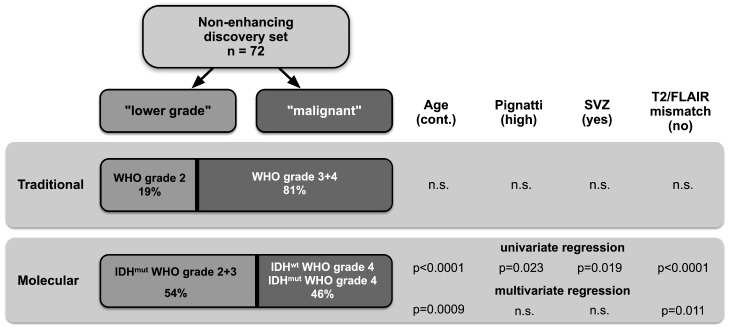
Malignancy was stratified by two different grading systems: (1) traditional grading (WHO grade 2 vs. WHO grade 3 + 4) and (2) molecular grading (IDH^mut^ WHO grade 2–3 vs. IDH^wt^ GBM WHO grade 4 + IDH^mut^ astrocytoma WHO grade 4). Molecular diagnostics allowed clinical and radiographic features to predict malignancy in a more robust manner than traditional grading.
